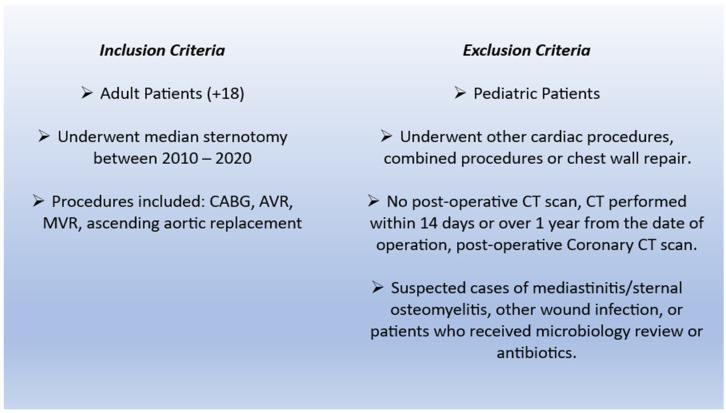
Inclusion and Exclusion Criteria. CT-computed tomography, CABG-coronary artery bypass grafting, AVR-aortic valve replacement, MVR-mitral valve replacement.
